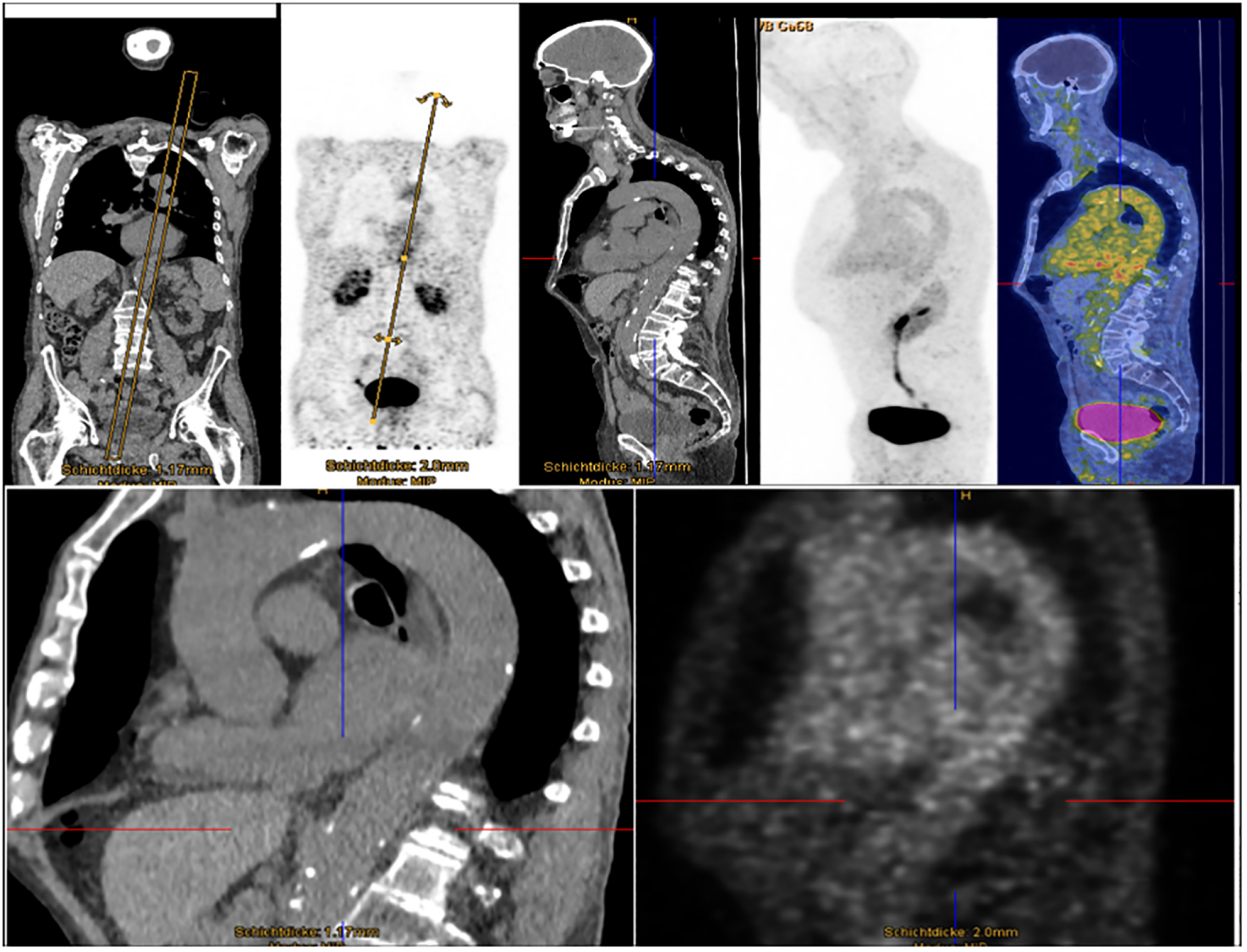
Enhanced [^68^Ga]Ga-DOTA-Siglec-9 uptake in aortic and subclavian regions. Whole-body PET images after intravenous injection of 120 MBq of [^68^Ga]Ga-DOTA-Siglec-9. Distribution of [^68^Ga]Ga-DOTA-Siglec-9 51 min after injection, based on imaging for one minute per bed position, revealed increased uptake in projection on the aortic and subclavian vessels and the heart. The increased uptake in the descending aorta diminishes abruptly while passing the diaphragm, without any caliber jump in the CT images.
